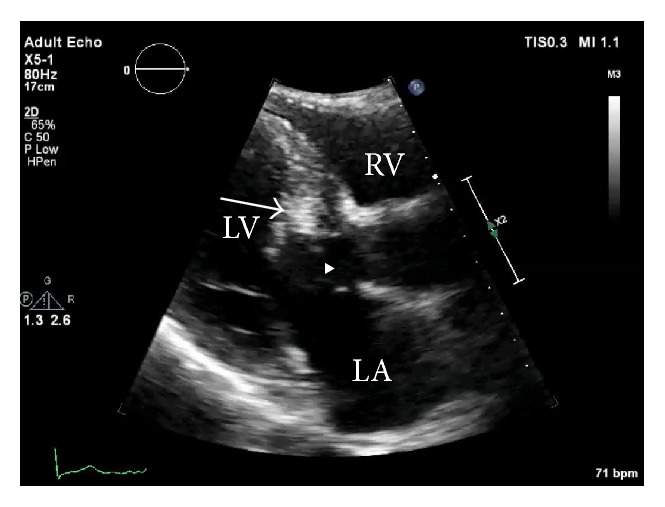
Preoperative Transthoracic Echocardiogram, Parasternal Long Axis. LA: left atrium; LV: left ventricle; RV: right ventricle; arrow: interventricular septal mass; arrow head: left ventricular outflow tract.
